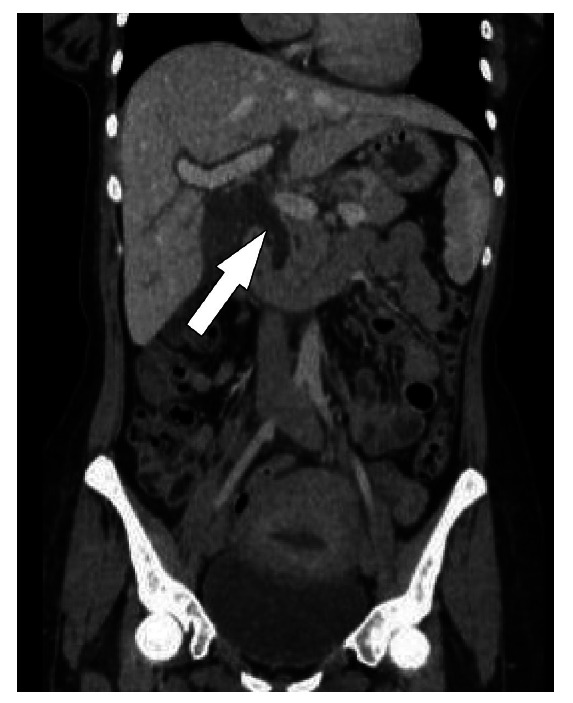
Computed tomography scan of a patient with common bile duct dilation (arrow).
